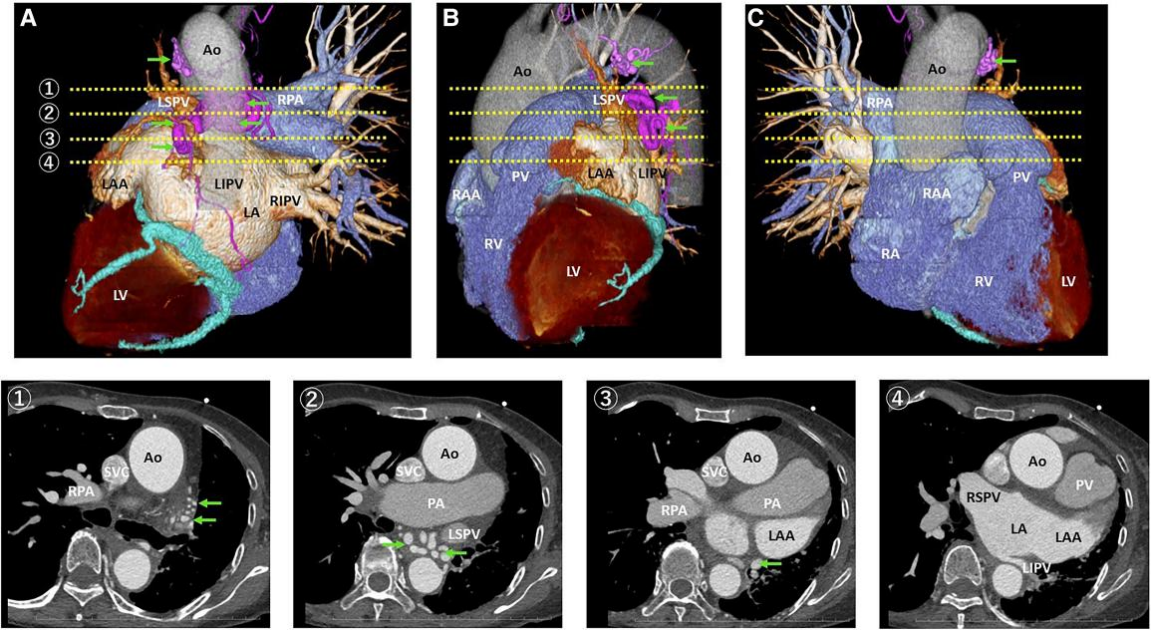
Three-dimensional computed tomography images (*A–C*) and axial slice (1–4). Arrows indicate systemic collateral vessels supplying the left lung. Ao = aorta; LSPV = left superior pulmonary vein; LIPV = left inferior pulmonary vein; RIPV = right inferior pulmonary vein; RPA = right pulmonary artery; PA = pulmonary artery; PV = pulmonary valve; LAA = left atrial appendage; RAA = right atrial appendage; LA = left atrium; RA = right atrium; LV = left ventricle; RV = right ventricle;.
